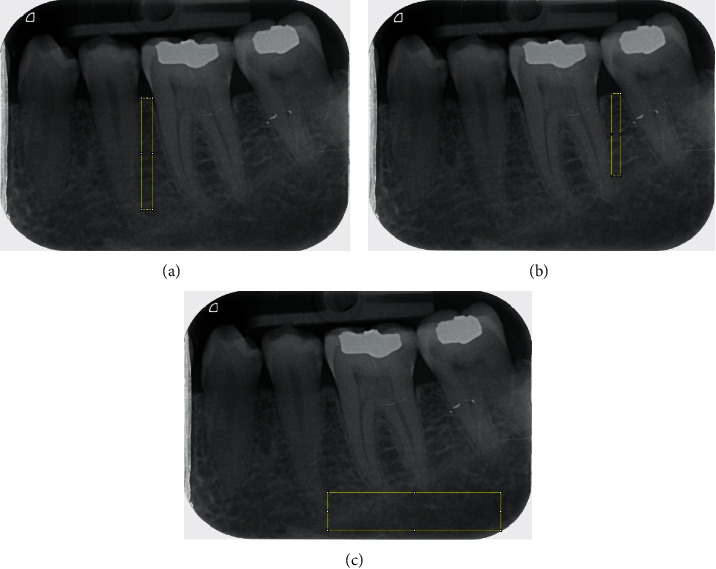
Selected regions of interest (ROIs) in periapical images: (a) mesial ROI, (b) distal ROI, and (c) apical ROI.
